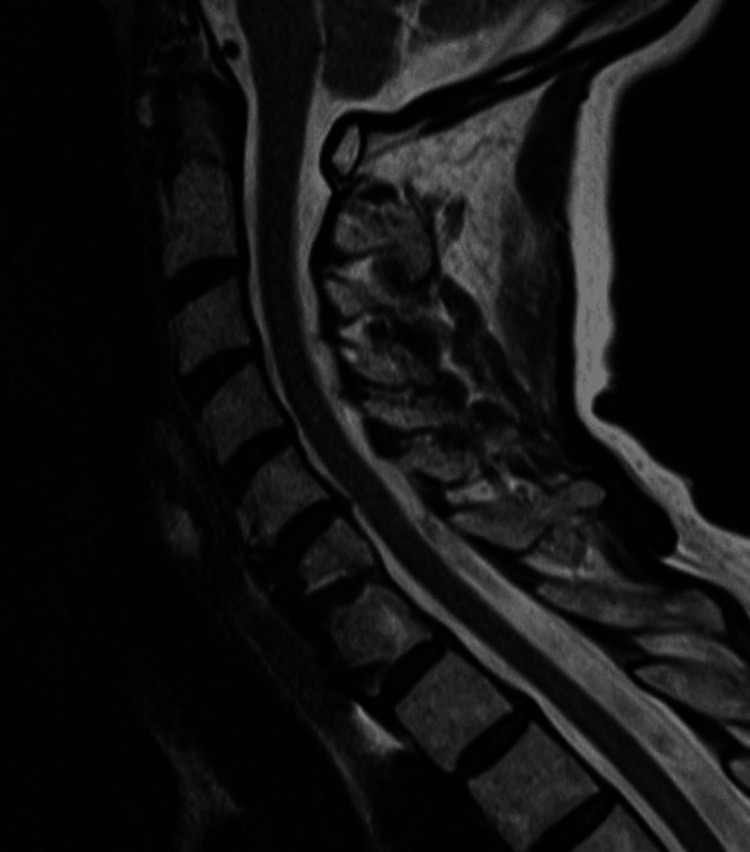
T2-weighted MRI of patient’s mid-sagittal cervical spine approximately two months before medial branch block injection.
